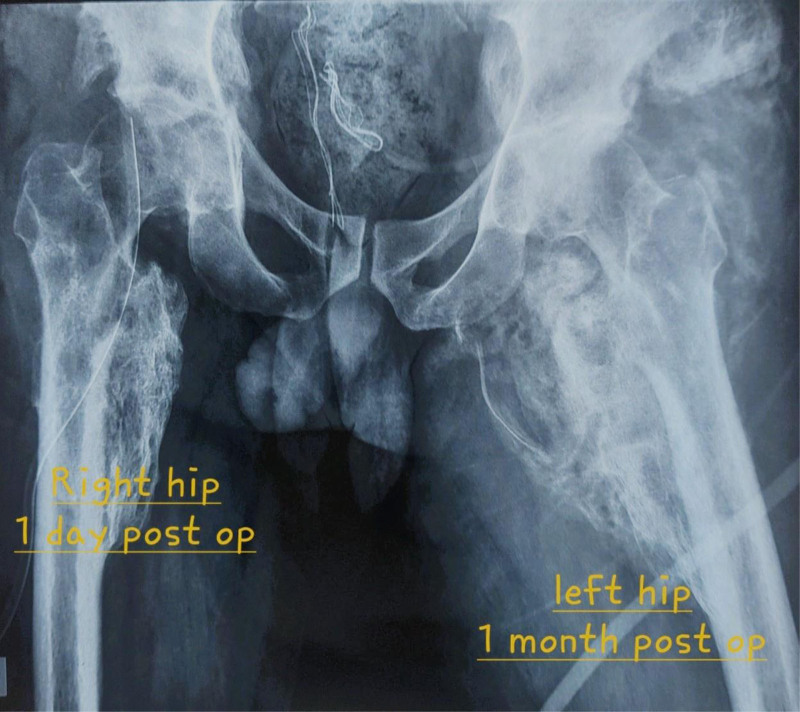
X-ray of the pelvis after the removal surgery of the osteochondroma in both hips.
